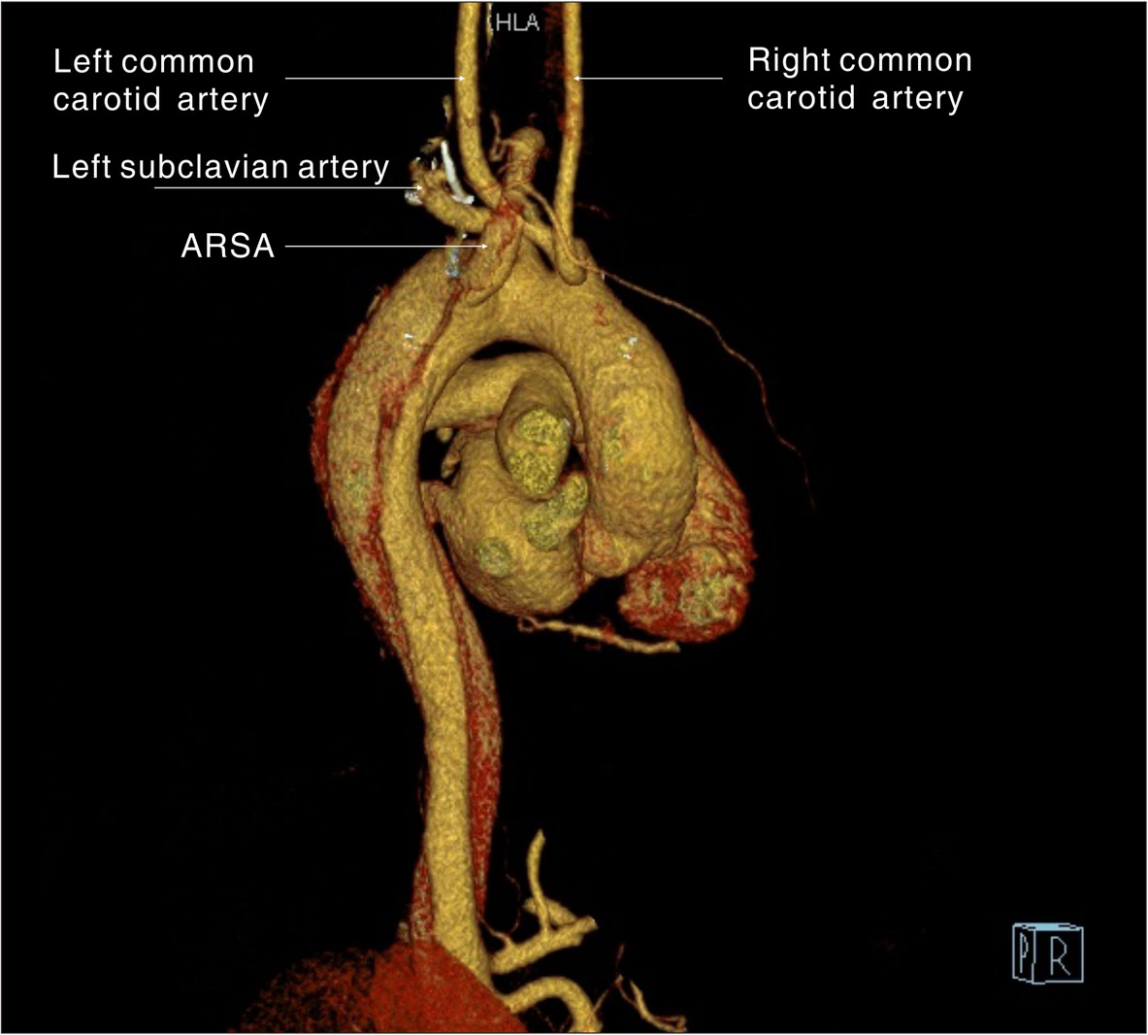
3D computed tomography reconstruction shows the aberrant right subclavian artery running distally to the left subclavian artery
